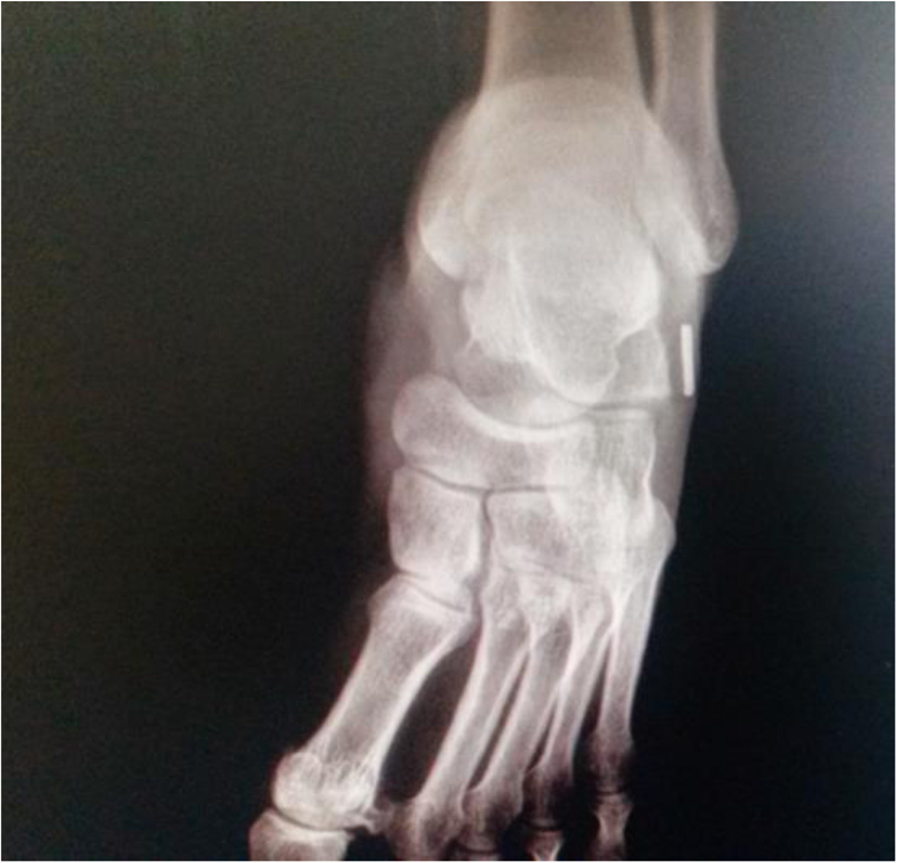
Anteroposterior plain radiography showing a Kirschner wire in the dorsolateral side of the right foot
